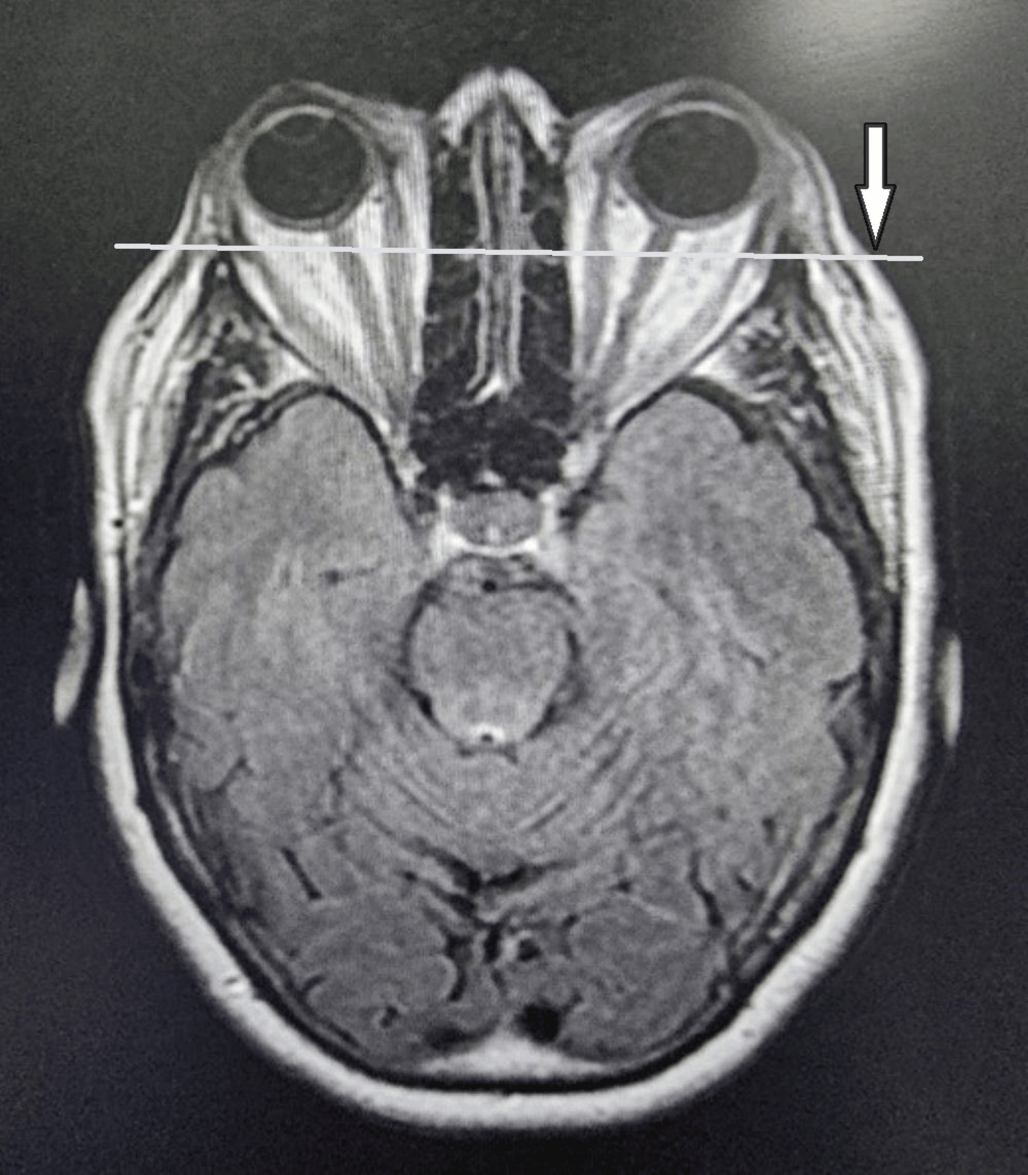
Orbital MRI axial view showing bilateral proptosis (white arrow) Image provided by the ENT, head and neck surgery department of Hassan II University Hospital of Fez, Morocco, taken during consultation
